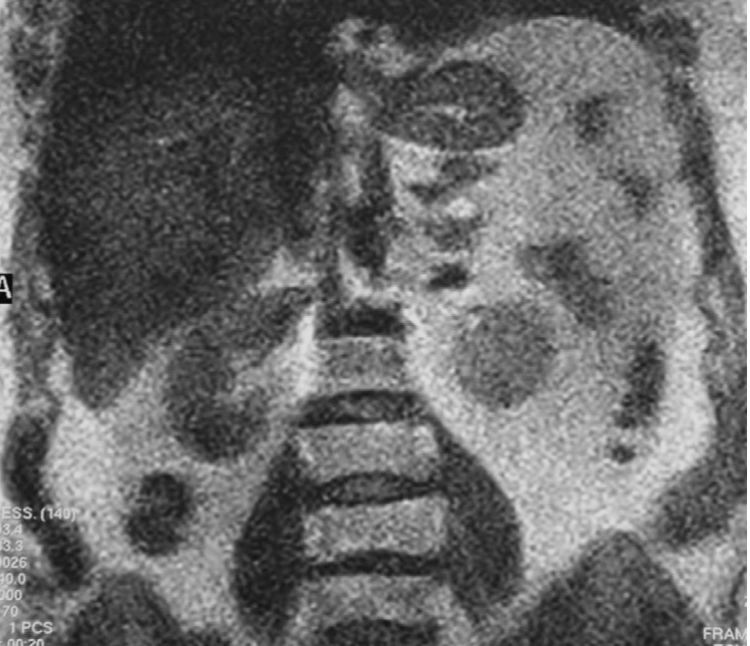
MRI T2-weighted imaging: little hyperintensive nodular lesion in the upper pole of the cystic lesion related to a daughter cyst.
